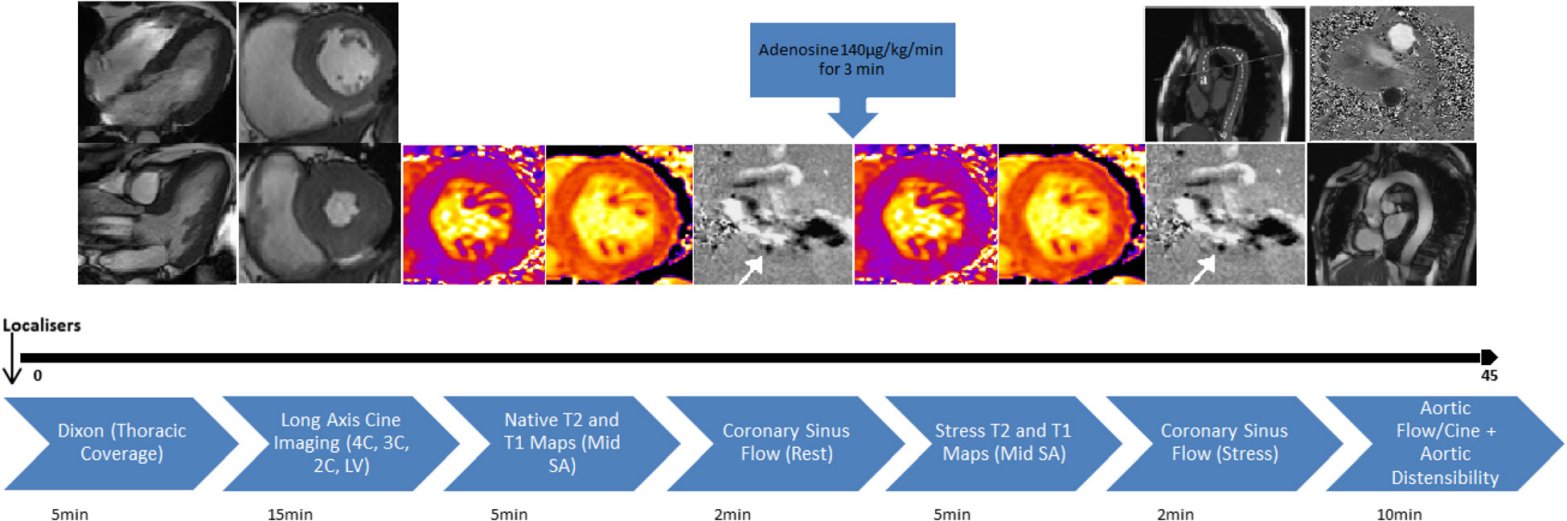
Cardiac MRI Protocol. (4/3/2C, 4/3/2 Chamber; LV, Left Ventricular; SA, Short Axis)
